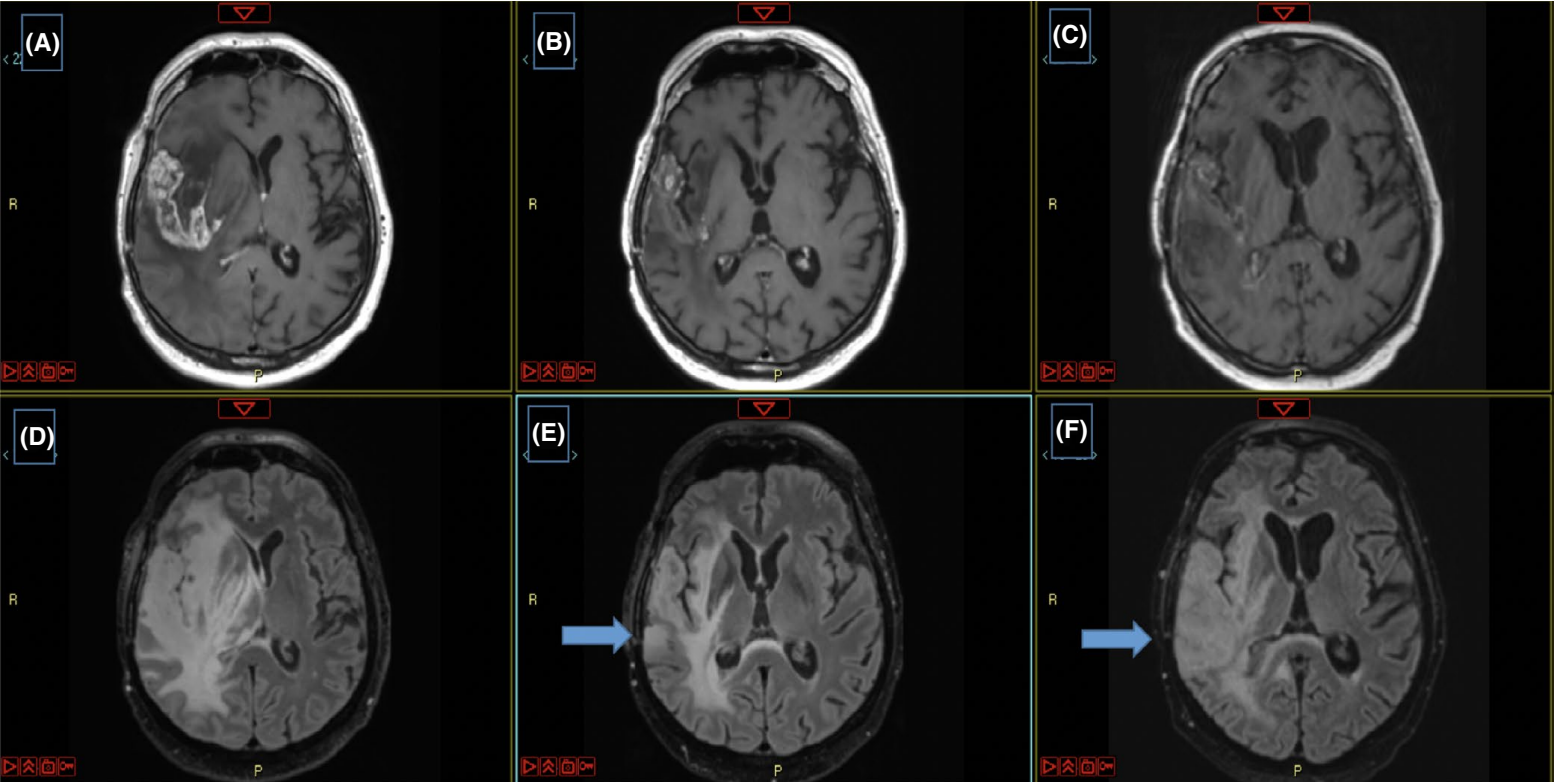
(A) T1 post‐contrast MRI before regorafenib treatment. (B) T1 post‐contrast MRI 3 months after starting regorafenib. (C) T1 post‐contrast MRI 6 months after starting regorafenib. (D) T2/FLAIR MRI before regorafenib treatment. (E) T2/FLAIR MRI 3 months after starting regorafenib. (F) T2/FLAIR MRI 6 months after starting regorafenib
